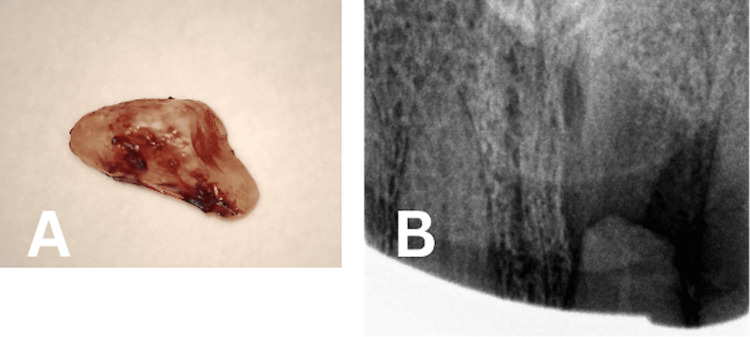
(A) Removed Apical Fragment and (B) Postoperative Radiograph After Apical Fragment Removal
